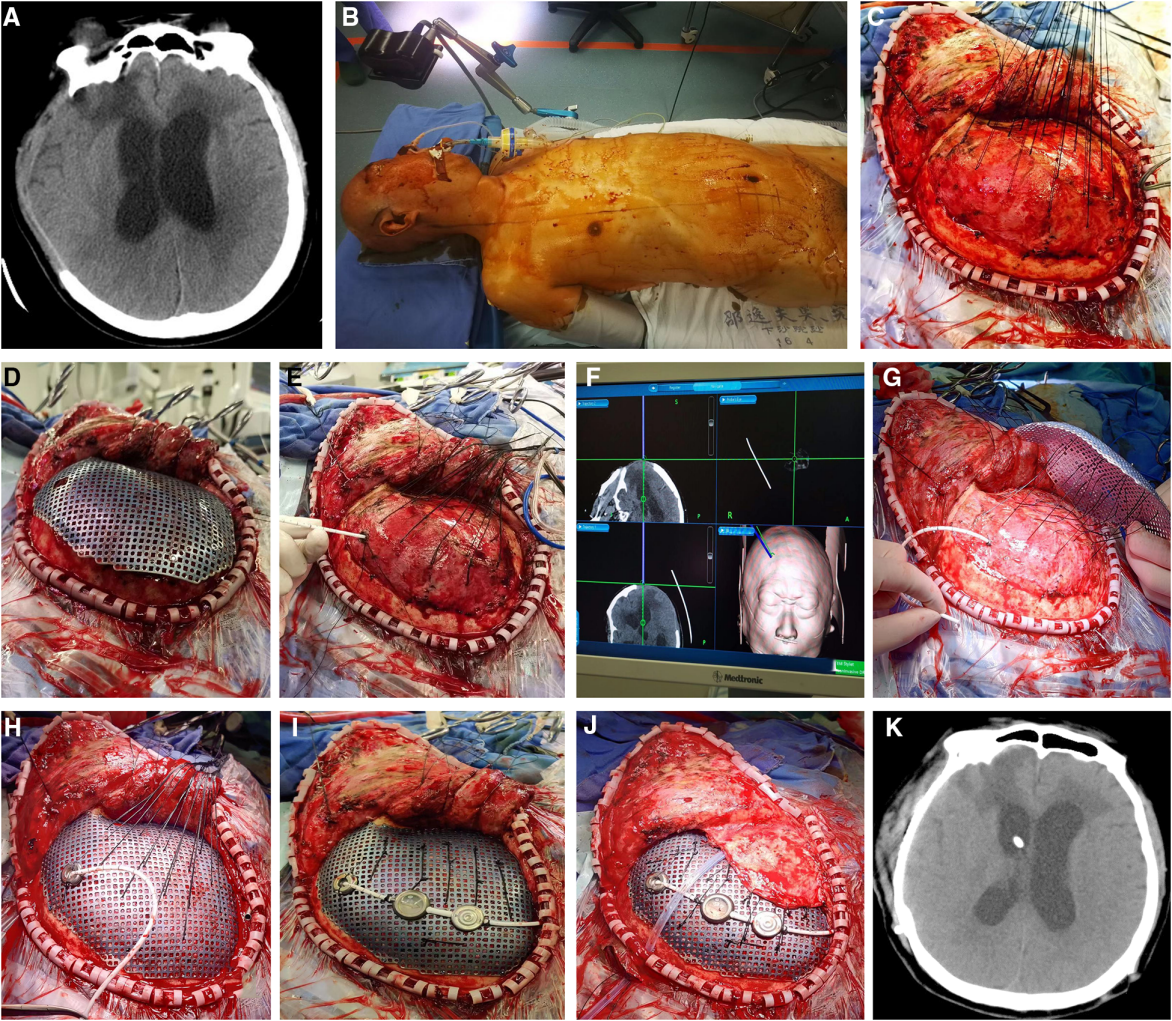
(A) Presurgical head CT scan demonstrated expansion of ventricular system, which was diagnosed as hydrocephalus. (B–J) Because of the encephalomeningocele, we first identified the entry point on the dura matter just close to the bone margin by the neuronavigation system. Then, ventricular puncture was performed to release the CSF till the titanium mesh could be attached to dura matter adequately, and parts of the shunting devices were further connected. (K) No operation-related complication was shown in the postsurgical radiological examinations. CSF, cerebrospinal fluid.
